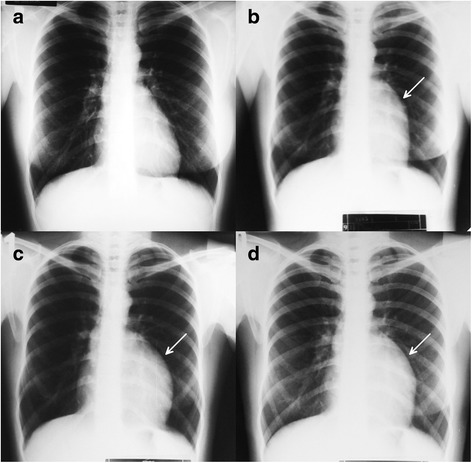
Chest X-ray examinations performed in 2004 (panel a), 2007 (panel b), 2010 (panel c) and in 2014 (panel d). *Arrows* show the enlarged cardiac silhouette
